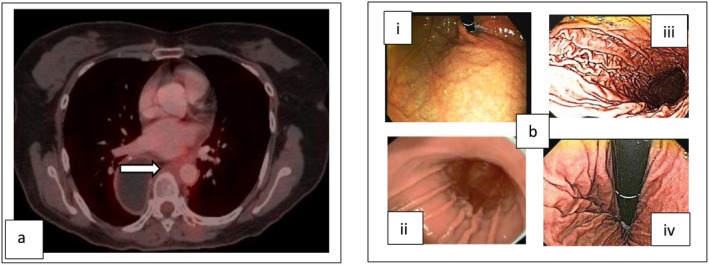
(a) White arrow in axial section of response PET‐CT after second time curative chemo‐radiotherapy shows complete metabolic response. (b) Picture shows UGIE after second time curative chemo‐radiotherapy. (i, ii) shows upper and middle gastric conduit respectively and (iii, iv) shows lower part of gastric conduit.
